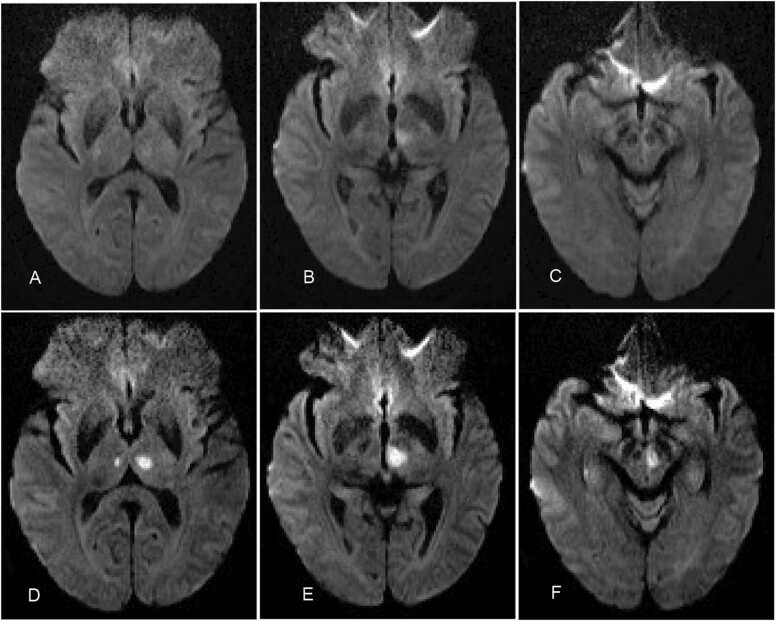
Diffusion-weighted MRI scans of Case 1 patient with AOP infraction. Axial diffusion-weighted images A–C showed high-intensity lesions in the paramedian region of the left thalamus seemed to involve the midbrain along the middle line; D–F reviewed clear diffusion-limited signals on the images in the left side of the midbrain and the paramedian thalamus after the third day after the onset of the disease.
